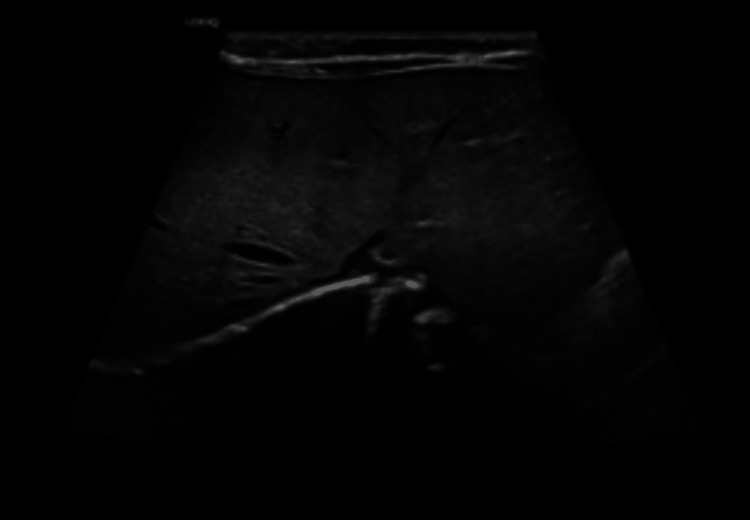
Postoperative ultrasound of the abdomen at the porta hepatis shows a normal liver with complete resection of the lesion.
